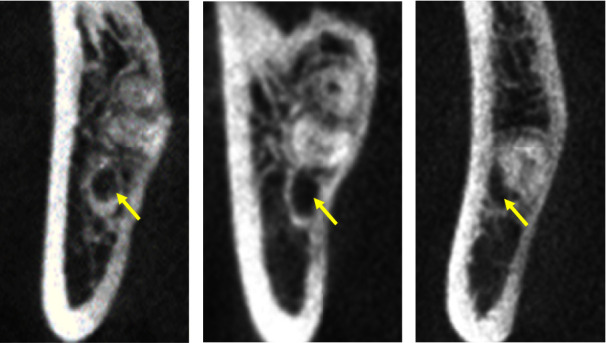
The CBCT image of the cortical bone state of the mandibular canal in the apical region of MTM. The yellow arrow represents the mandibular canal. (a) The root of the MTM and the cortical bone of the mandibular canal is intact without contact; (b) the root of the MTM is in contact with the intact cortical bone of the mandibular canal; (c) the root of the MTM is in contact with the disrupted cortical bone of the mandibular canal.
